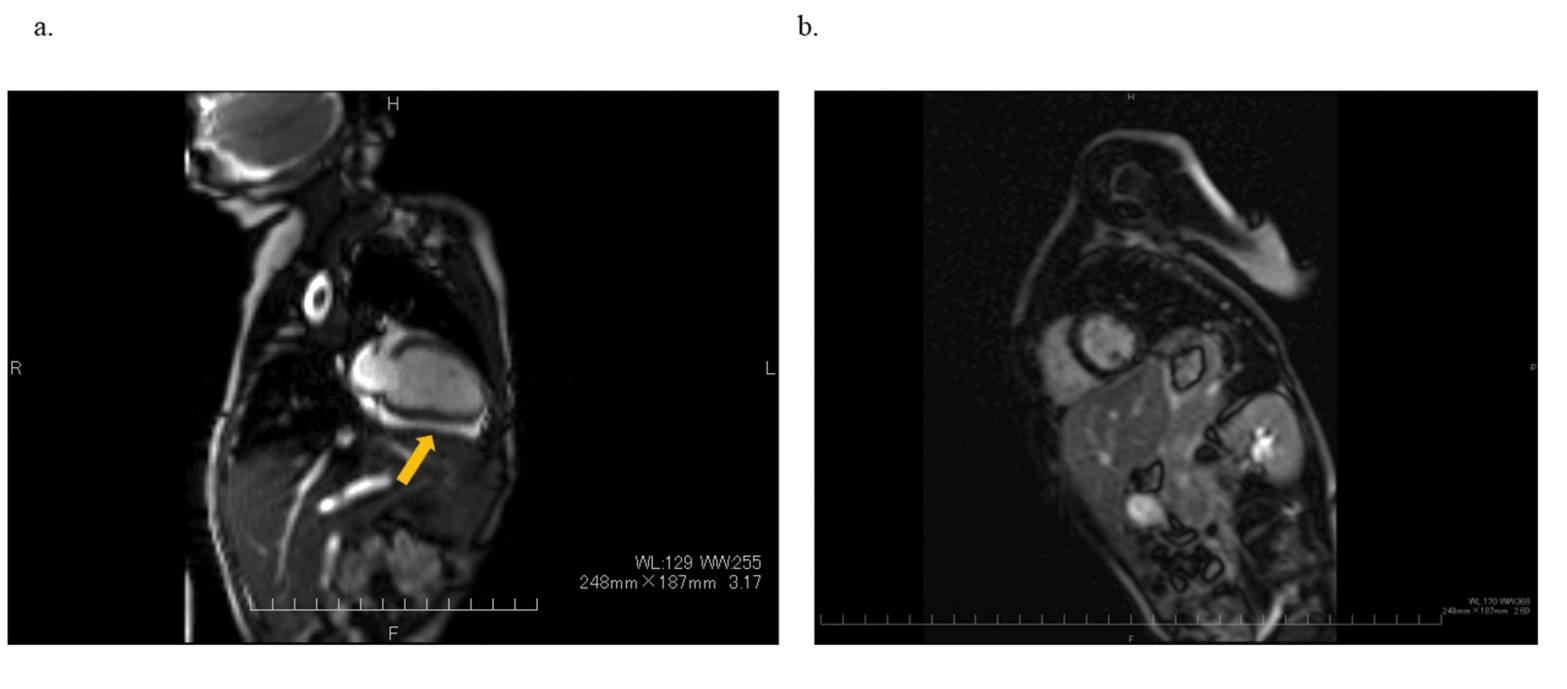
Cardiac MRI MR: magnetic resonance; LGE: late gadolinium enhancement; SSPF: steady-state free precession A cardiac MRI obtained after the recurrence of pericardial effusion demonstrates the presence of pericardial effusion without any evidence of LGE in the myocardium or pericardium. (a) SSPF image depicting pericardial effusion and the absence of myocardial edema. (b) The LGE image displays no enhancement in the myocardium or pericardium
